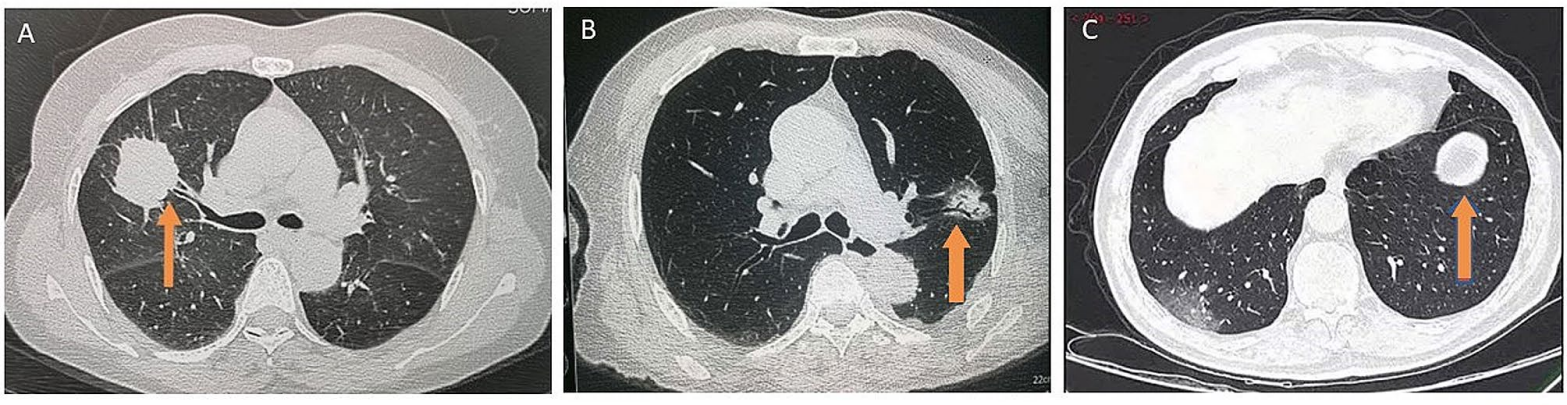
CT imaging shows (A) the presence of bronchus truncation sign (B) the presence of bronchus passage sign (C) no bronchus sign
